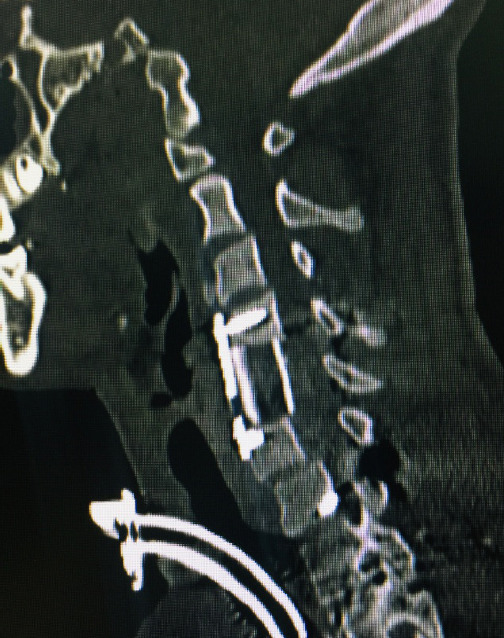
One month postoperative computed tomography sagittal section showing anterior cervical plate and screws at C4 and C6 with fibular graft in between.
